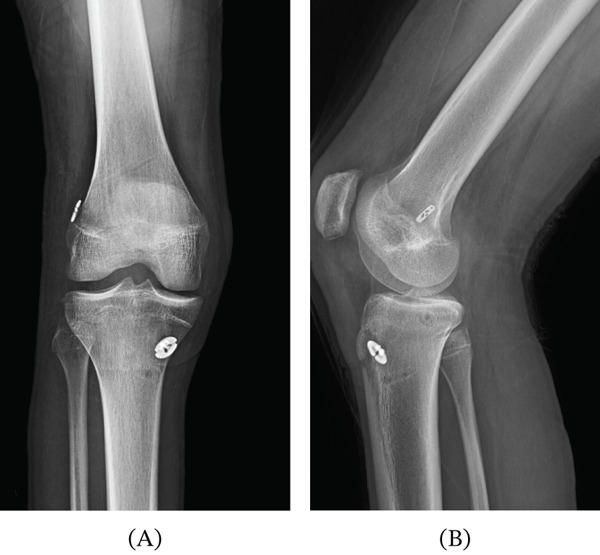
Postoperative radiographic evaluation following ACL and ALL reconstruction. Postoperative anteroposterior and lateral radiographs of the right knee demonstrating appropriate positioning of fixation devices following anterior cruciate ligament reconstruction with internal brace augmentation and anterolateral ligament reconstruction. No evidence of hardware complication or malalignment is observed.
